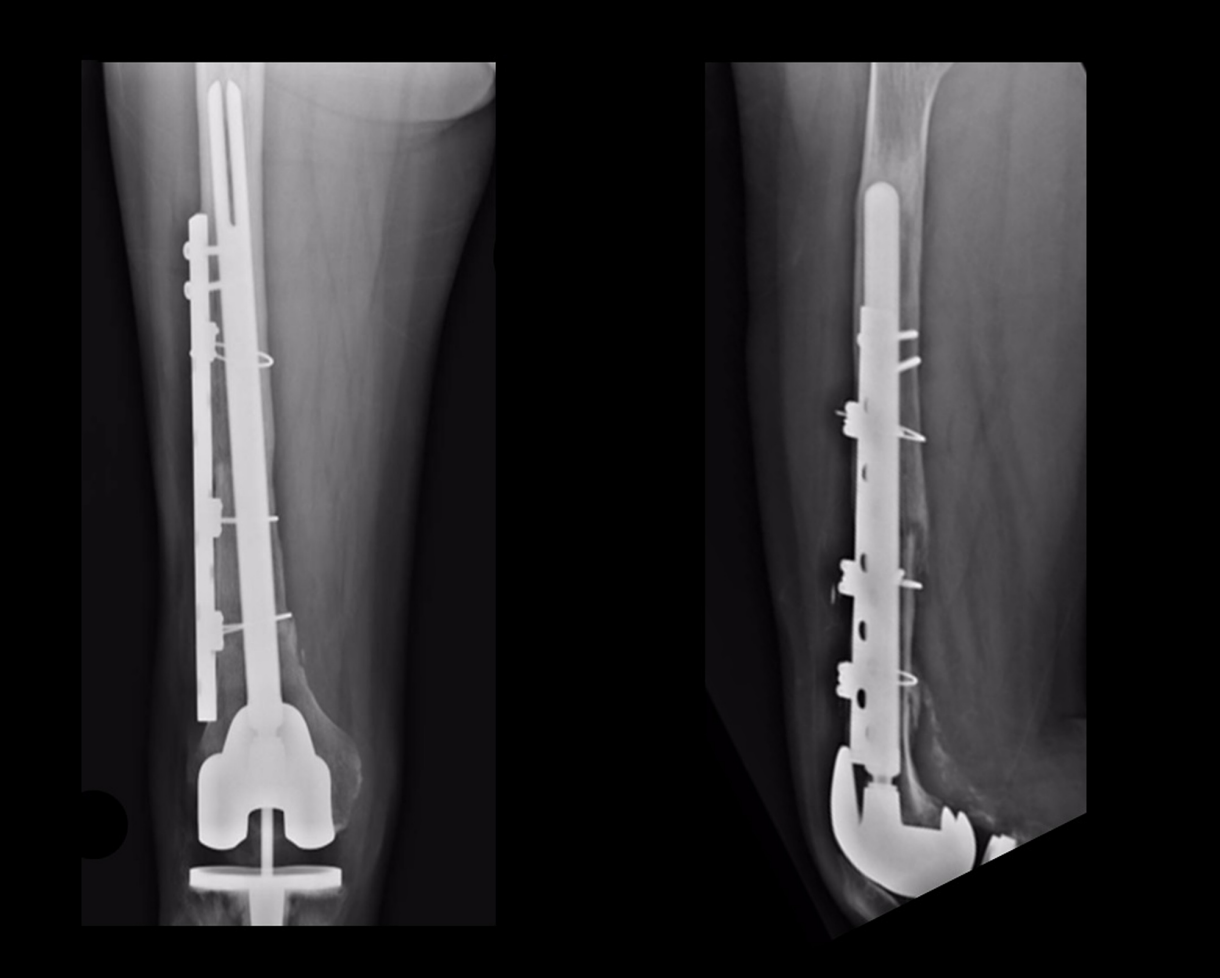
Anteroposterior and lateral X-rays of the femur taken 10 years after allograft revision. The allograft is integrated, stable, and well fixed. No pain is reported by the patient.
